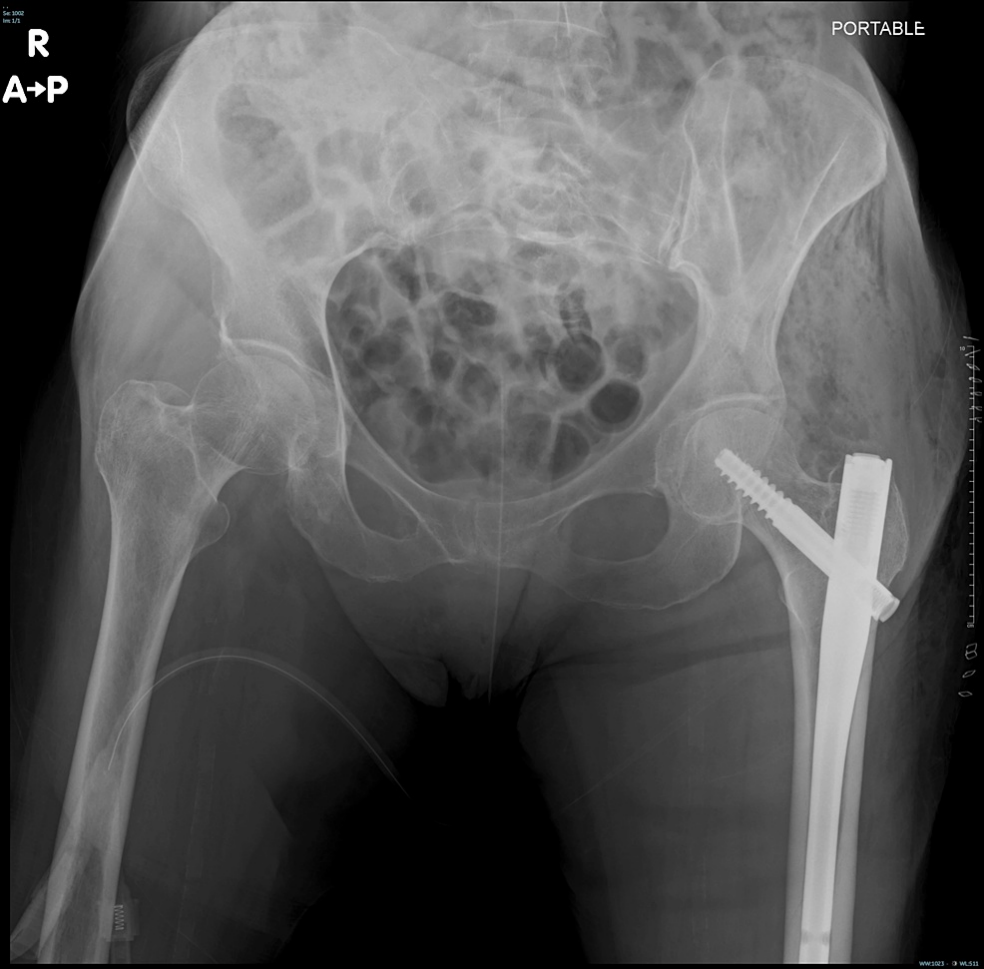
Plain radiograph taken immediately after surgery shows internal fixation of the left femoral neck with an intramedullary nail and screw. No other definite recent fracture lines are seen
